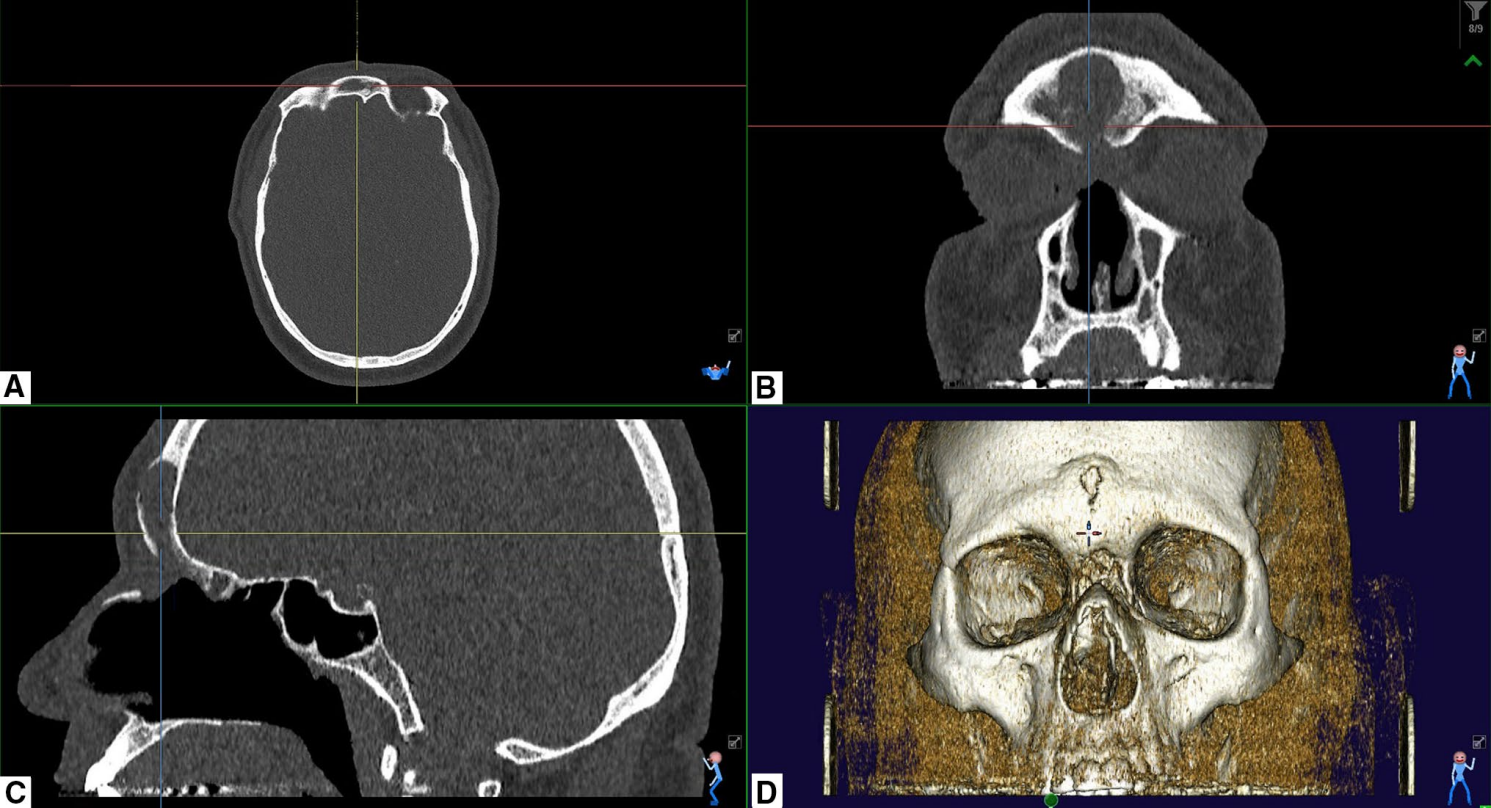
Post-operative CT scan of the 59-year-old male, 9 months after Draf III. Kuhn type IV cell has been opened and is not visible at the CT scan. There is residual inflammation in the frontal sinus which is compatible with the underlying chronic rhinosinusitis. A wider sinonasal frontal cavity has been obtained. a Axial, b coronal and c sagittal view. d 3D reconstruction
